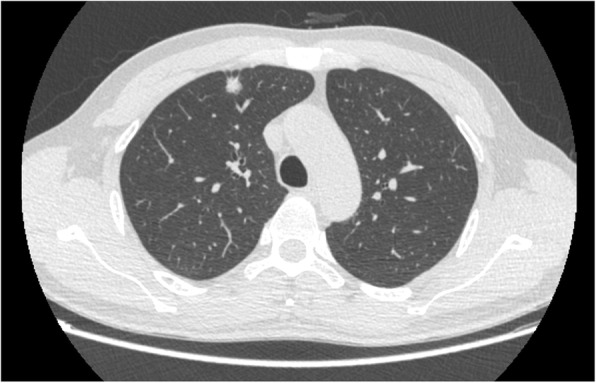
CT image showing a solid pulmonary nodule in the right upper lobe
